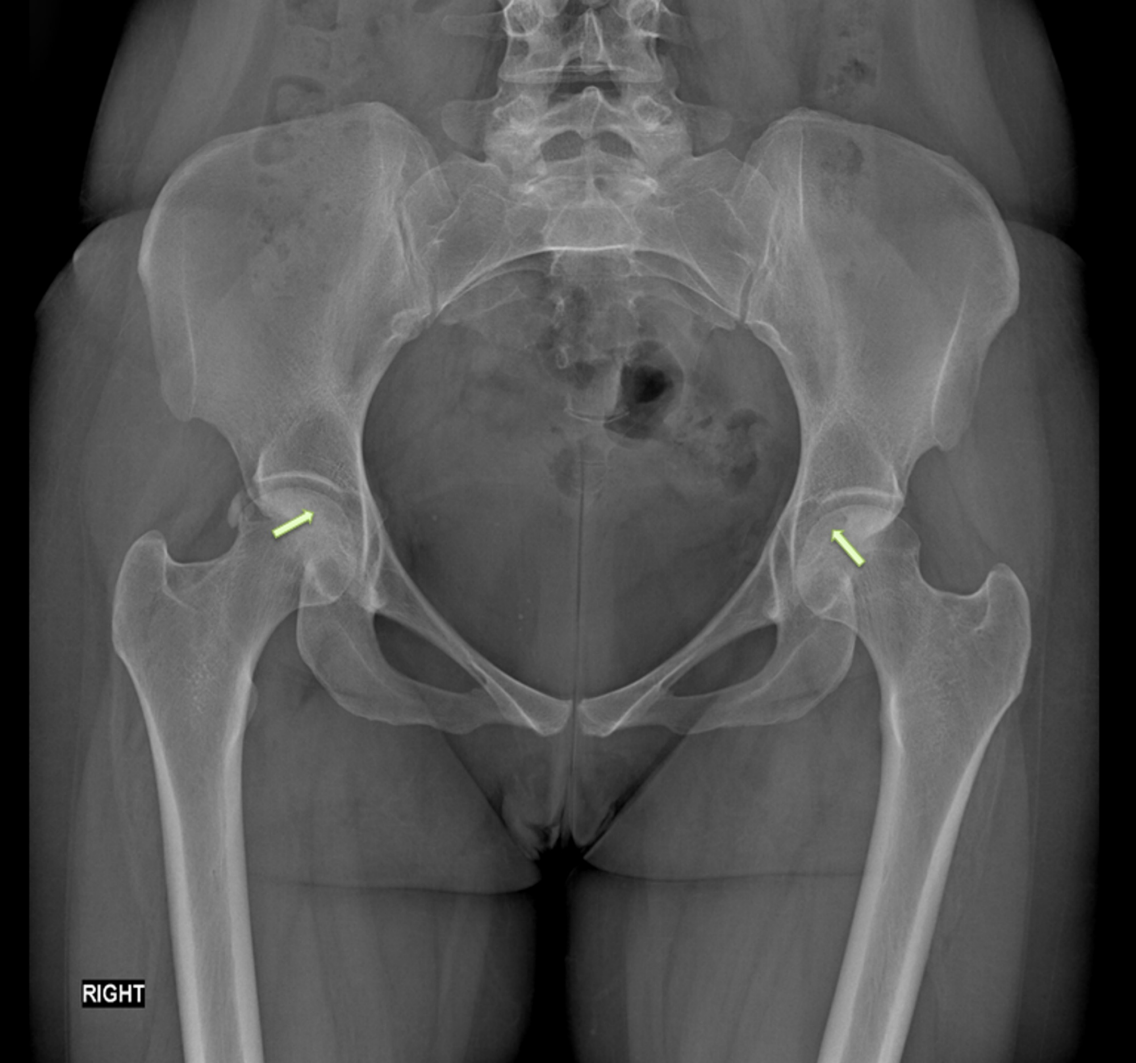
AP radiograph of the pelvis showing crescent signs AP: anteroposterior
